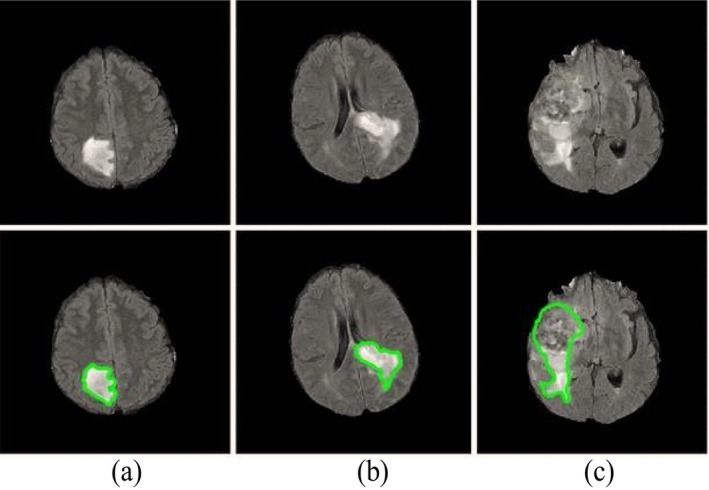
Examples of manual segmentation of brain tumors in MRI images. The first row displays the original images, and the second row shows the manually segmented brain tumor [28].
